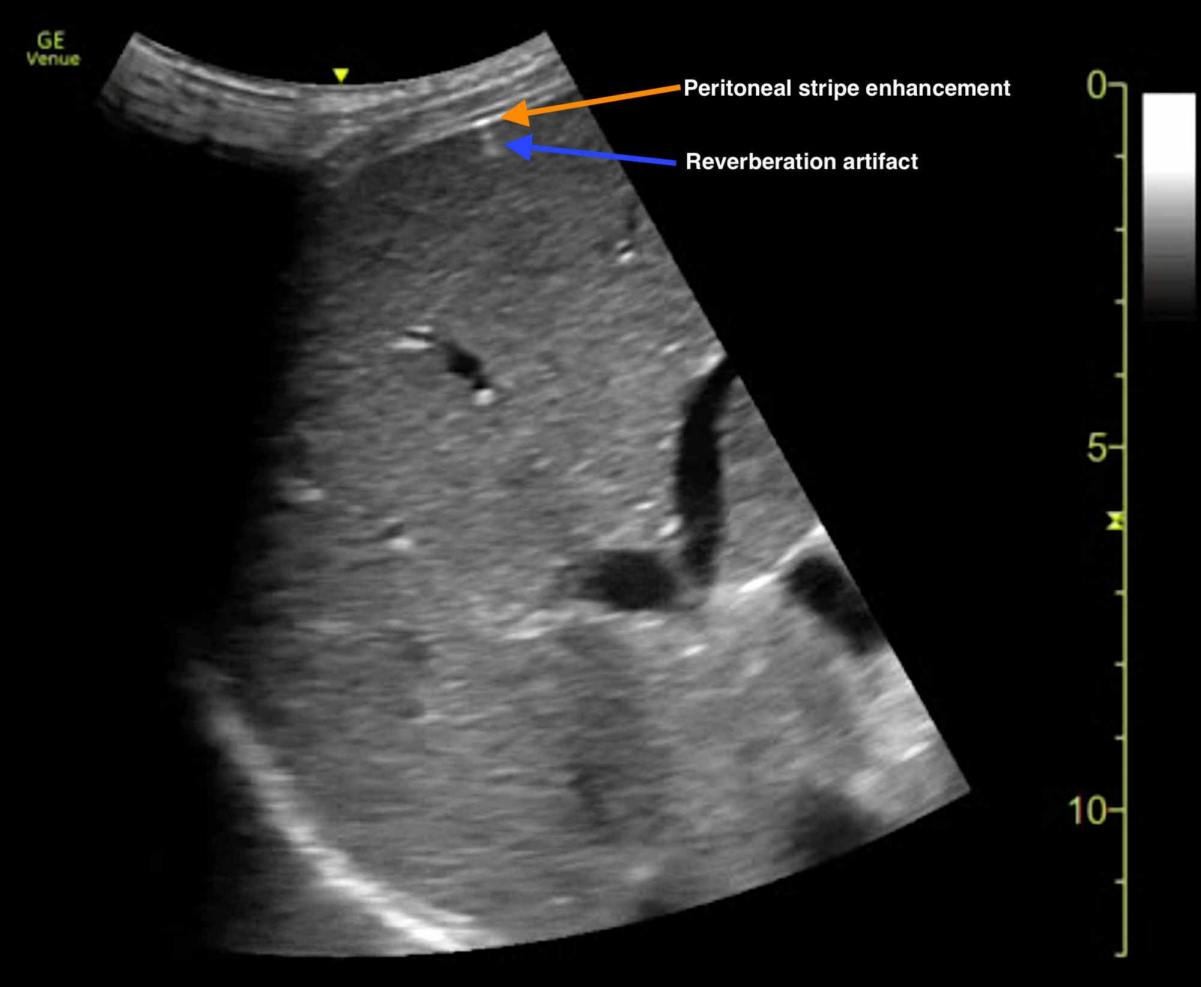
Point-of-care ultrasound diagnosis of pneumoperitoneum. Gas bubble abutting the liver and abdominal wall is indicated by peritoneal stripe enhancement (orange arrow) with corresponding reverberation artifact (blue arrow).
